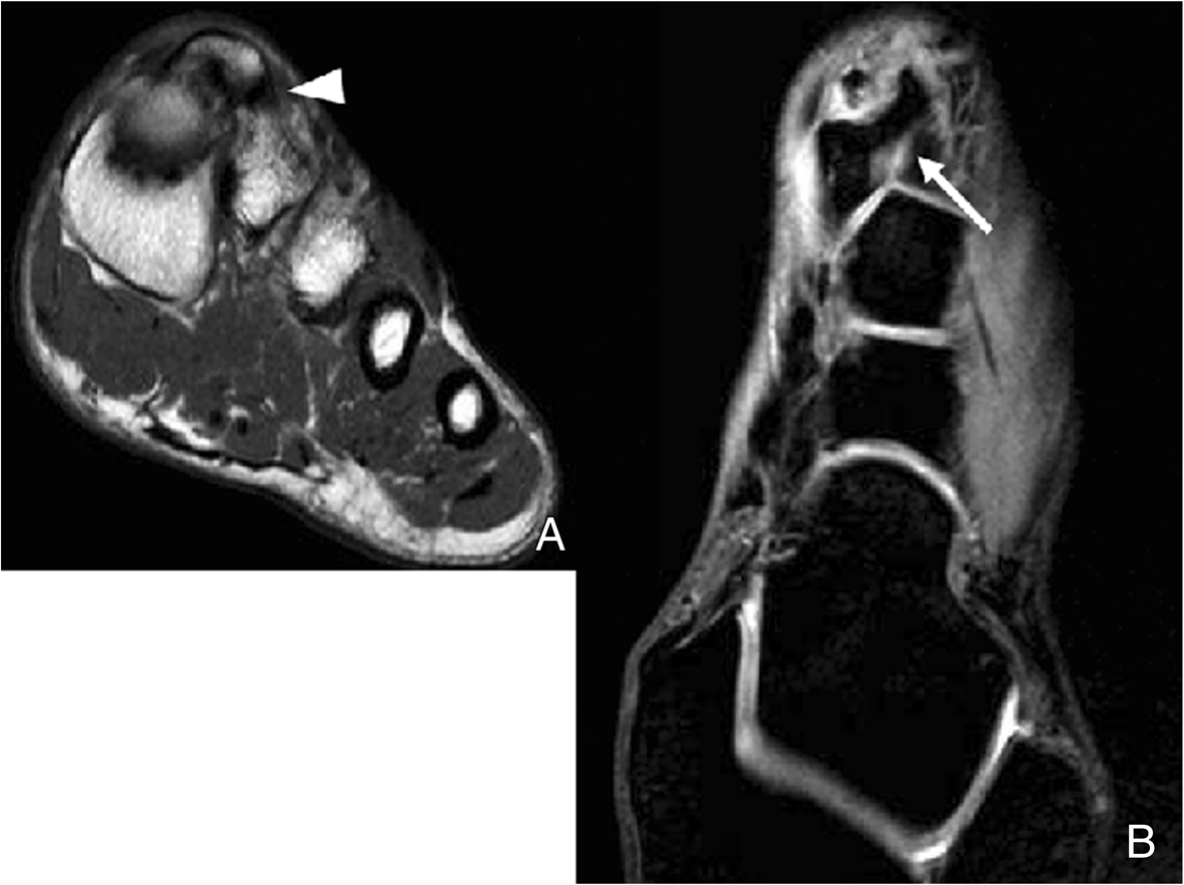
Os intermetatarseum. A 36-year-old man, investigated for pain in the Achilles. Radiographs demonstrate a spur projection in the dorsum of the foot, overlying the base of the metatarsal (not shown). a Coronal FSE T1 demonstrates how this articulates with the base of the second metatarsal, with a synchondrosis (white arrowhead). b Axial three-dimensional gradient echo water selective/fluid (WATSf) demonstrates this exostosis arises from the base of the first metatarsal, and extends over the joint with the base of the second metatarsal (white arrow). These ossicles rarely represent a cause or pathology, unless compression irritates the superficial and deep peroneal nerves
